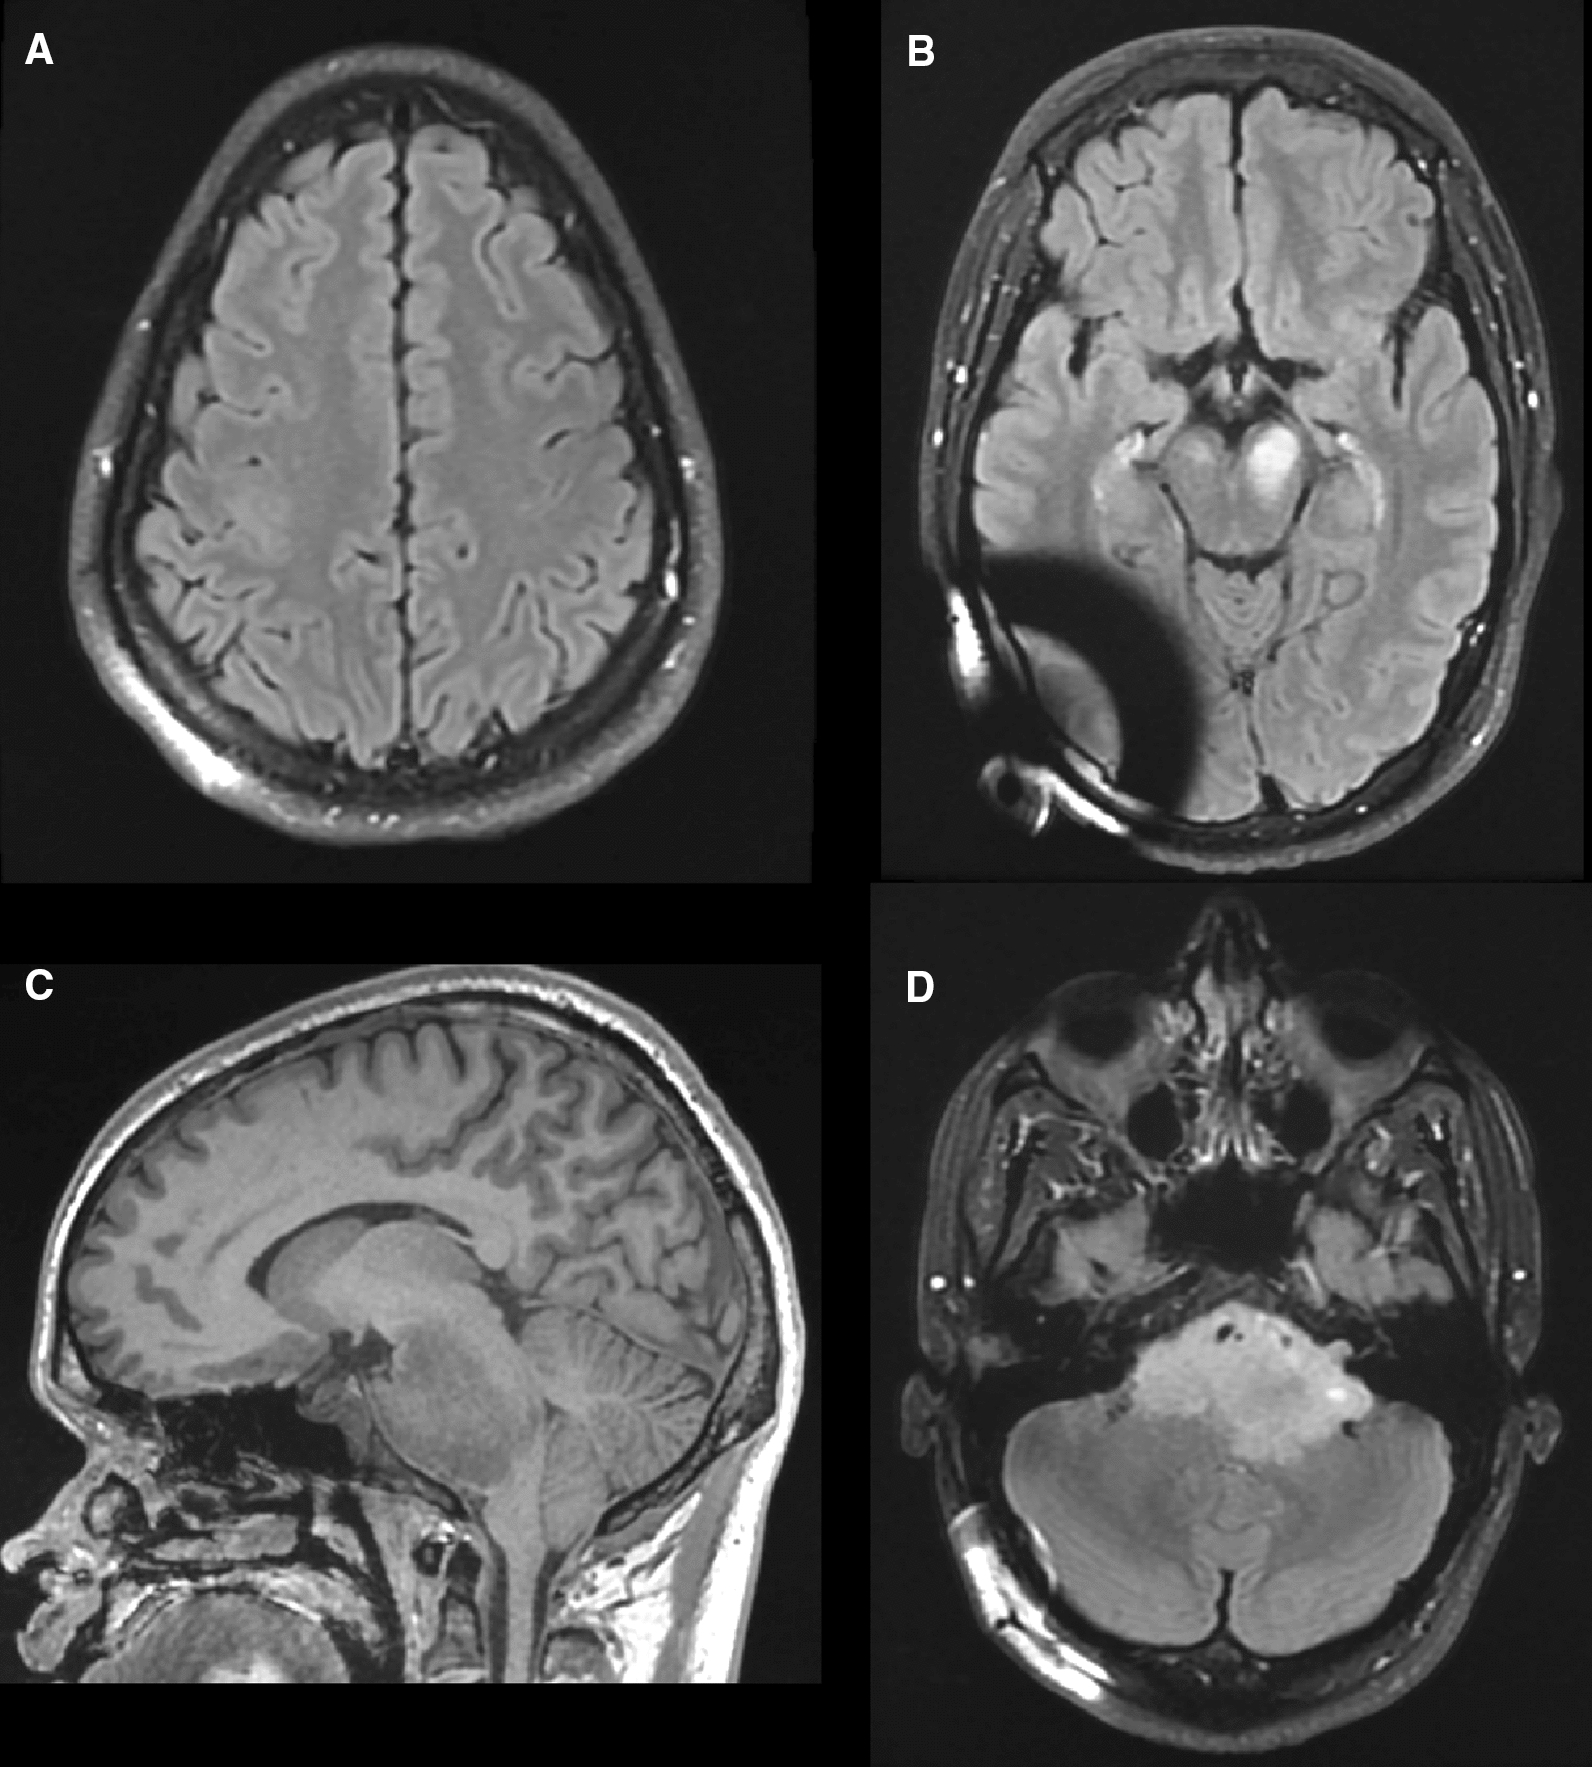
Follow up MRI following four cycles of PCV. A Hyperintense lesion of similar size and appearance in the precentral gyrus on axial T2-FLAIR. B Homogenous hyperintense lesions in the left and right cerebral peduncles on axial T2-FLAIR. C Large, primarily hypointense pontine lesion on sagittal T1 measuring 6.1 × 4.8 × 3.9 cm. Note herniation of the cerebellar tonsil. D Axial T2-FLAIR demonstrating new hyperintense foci along the left anterior base of the lesion that extends into the cerebellopontine cistern and internal auditory canal. Again, encasement of the basilar artery is noted but flow is patent
